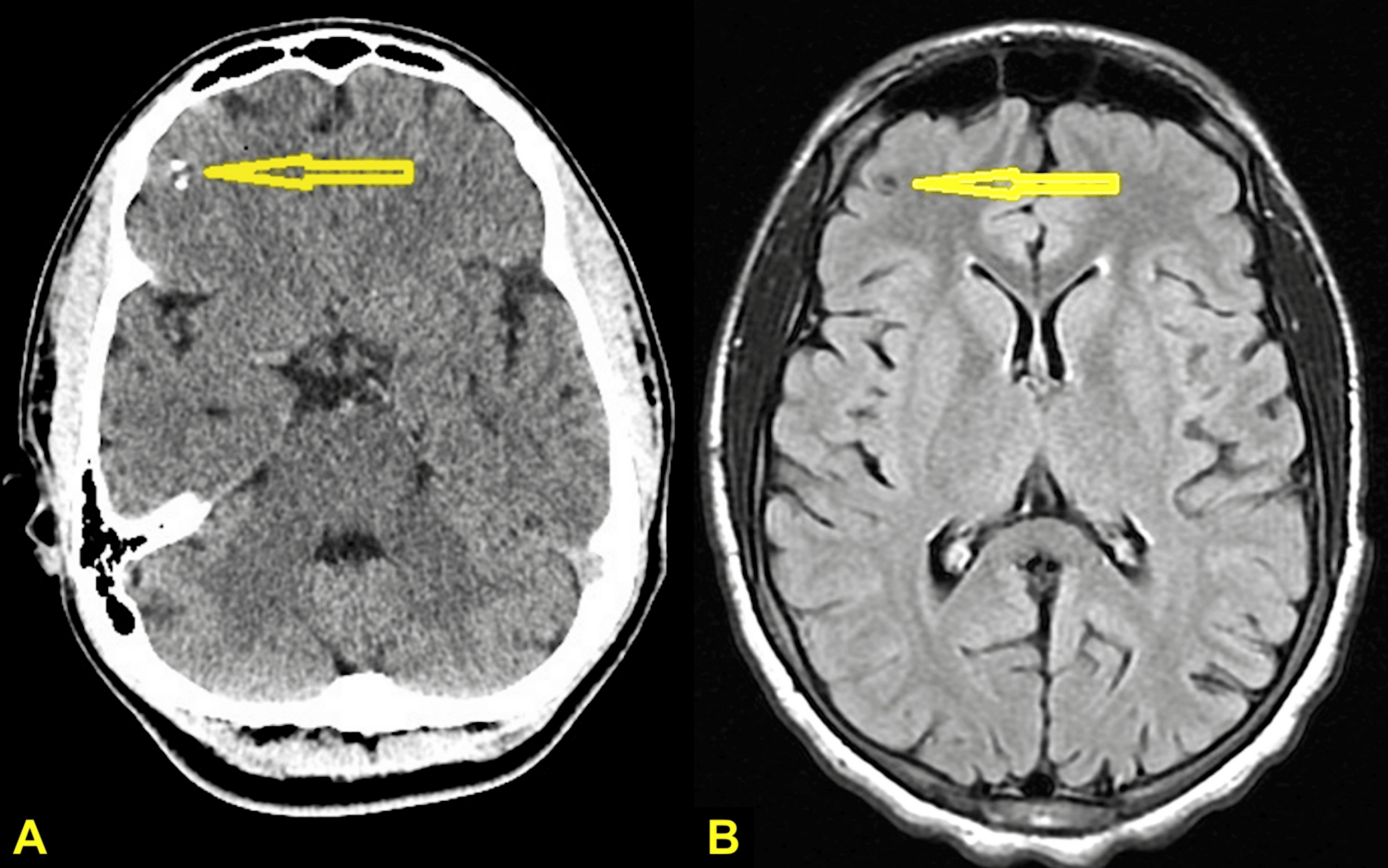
(A) A follow-up CT scan reveals a stable cystic lesion with partial calcification in the right frontal lobe. (B) An MRI from the same period confirms no significant changes, with a persistent stable cystic lesion in the right lateral frontal lobe
